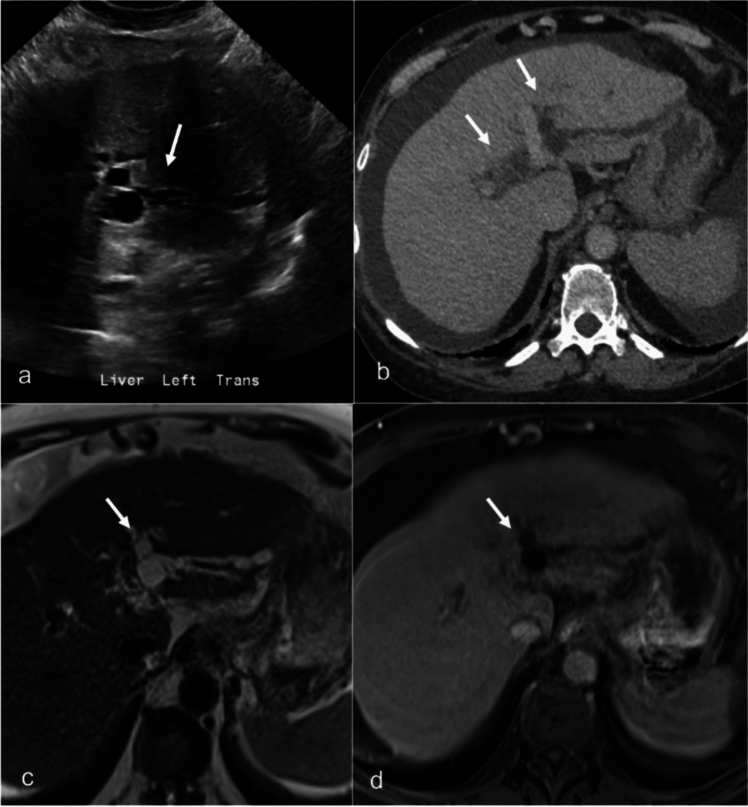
Intraductal papillary neoplasm of the bile duct in a 56-year-old female patient with alcoholic cirrhosis. Grayscale ultrasound images (a) shows biliary duct dilation (arrow). Axial CT with contrast (b) shows similar finding as US. Axial MRI T2-weighted fat-saturated (c) and postcontrast T1 (d) images show aneurysmal dilatation of the bile duct (arrows) with distal ductal dilatation. There is no proximal bile duct dilatation (not shown), and no intraduct mass is present. The surgical pathology confirmed the diagnosis
